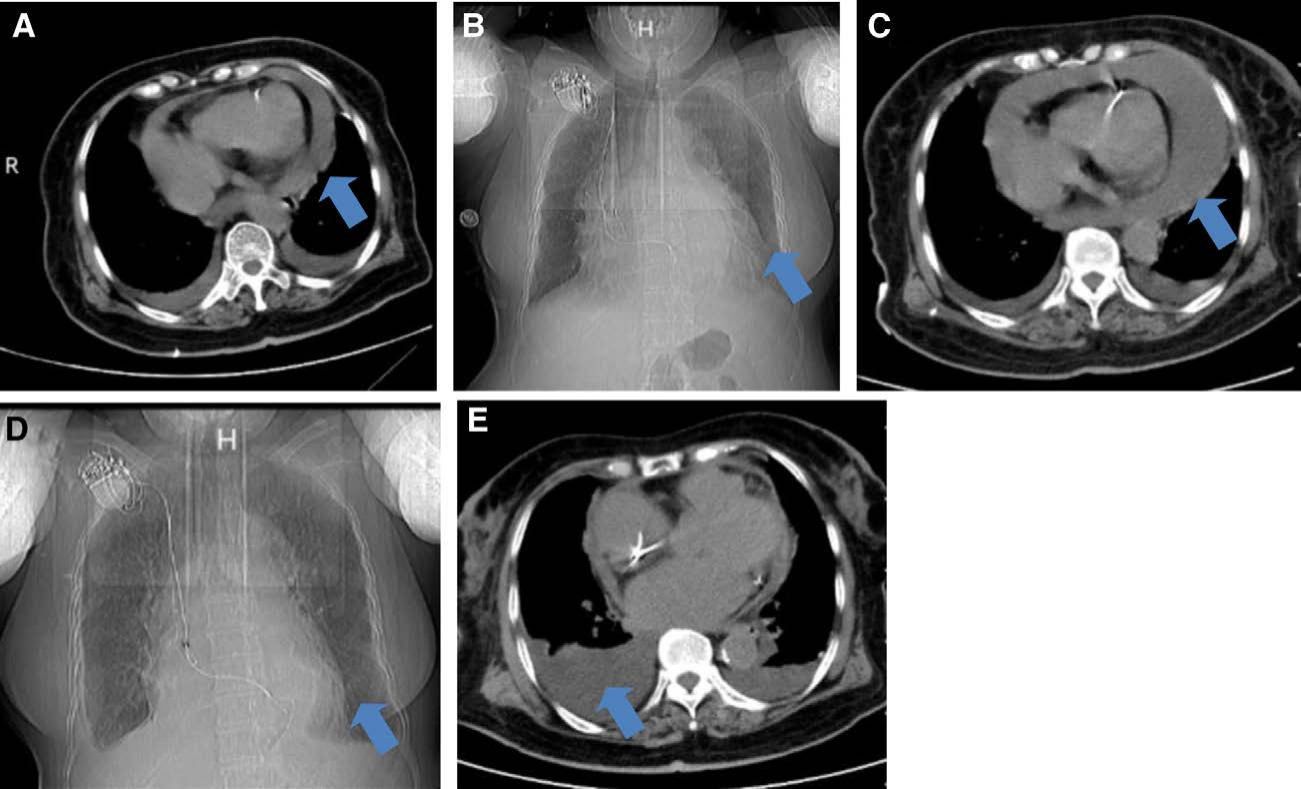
(A and B) First hospitalization chest CT showing PE and pleural effusion; (C) significant increase in pericardial effusion upon readmission. (D and E) Third hospitalization chest CT showing significant reduction in PE and right-sided pleural effusion. CT = computed tomography, PE = pericardial effusion.
